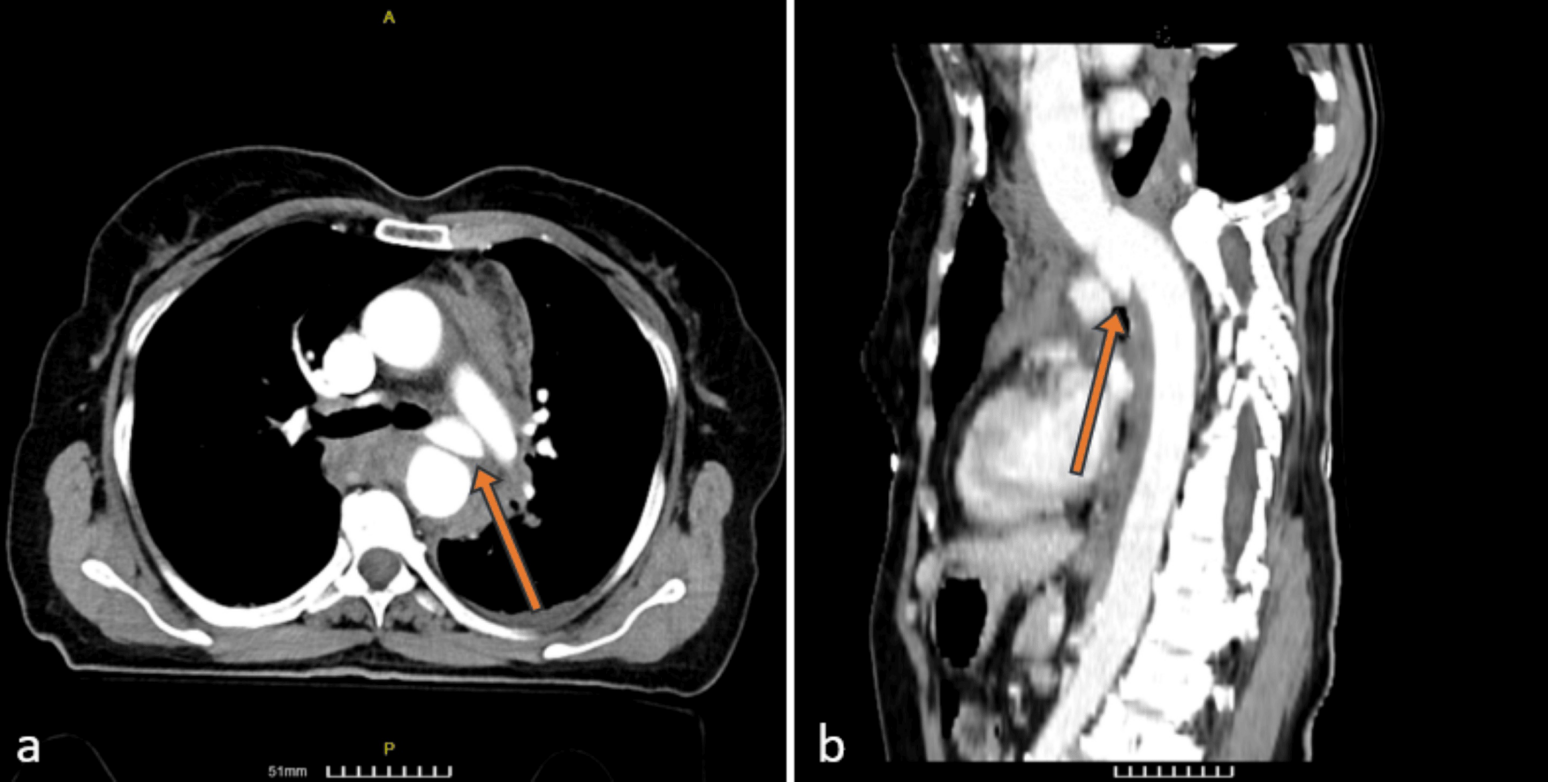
CT before intervention: (a) transverse view and (b) sagittal view The arrow indicates the rupture site at the level of the isthmus.
